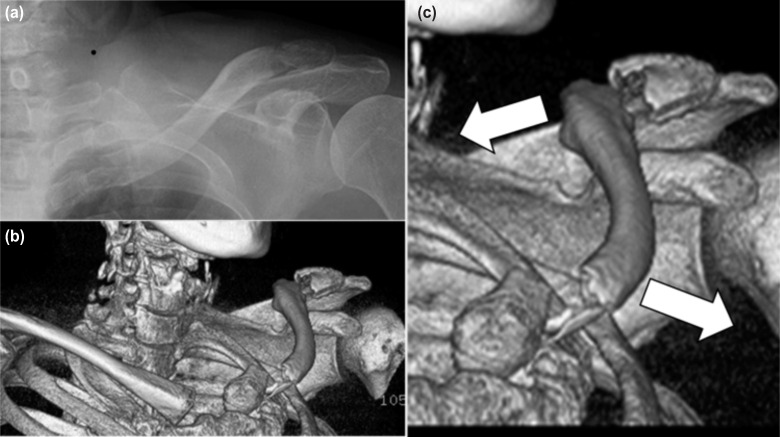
(a) Preoperative standard radiograph, (b) Preoperative three-dimensional CT scan image, (c) Proximal end of the clavicle was displaced anteriorly and the distal end posteriorly.
